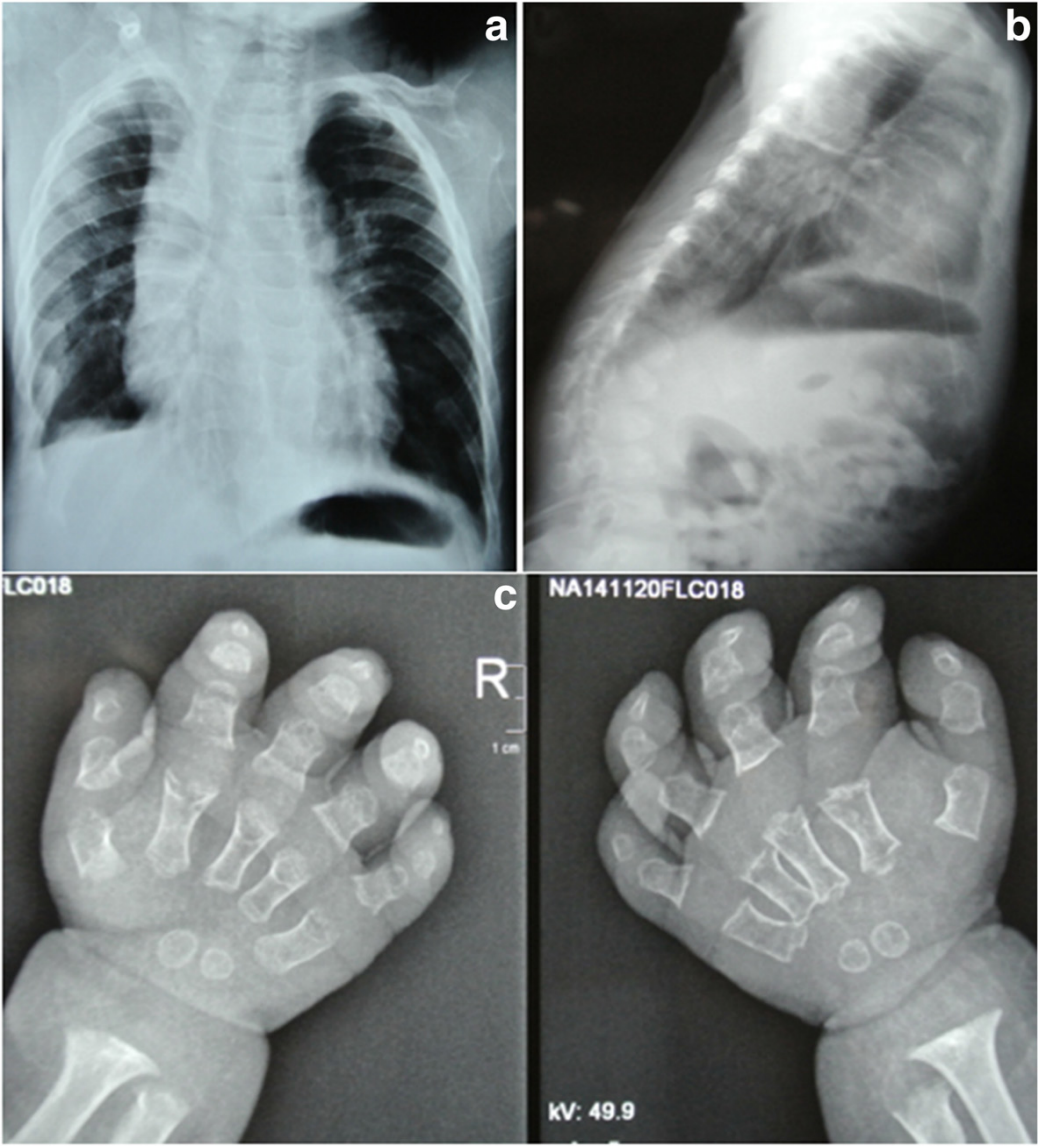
Spine X-ray images showed mild kyphosis (a) and scoliosis (b) at the thoracic and lumbar region. Bone age is delayed (c)
